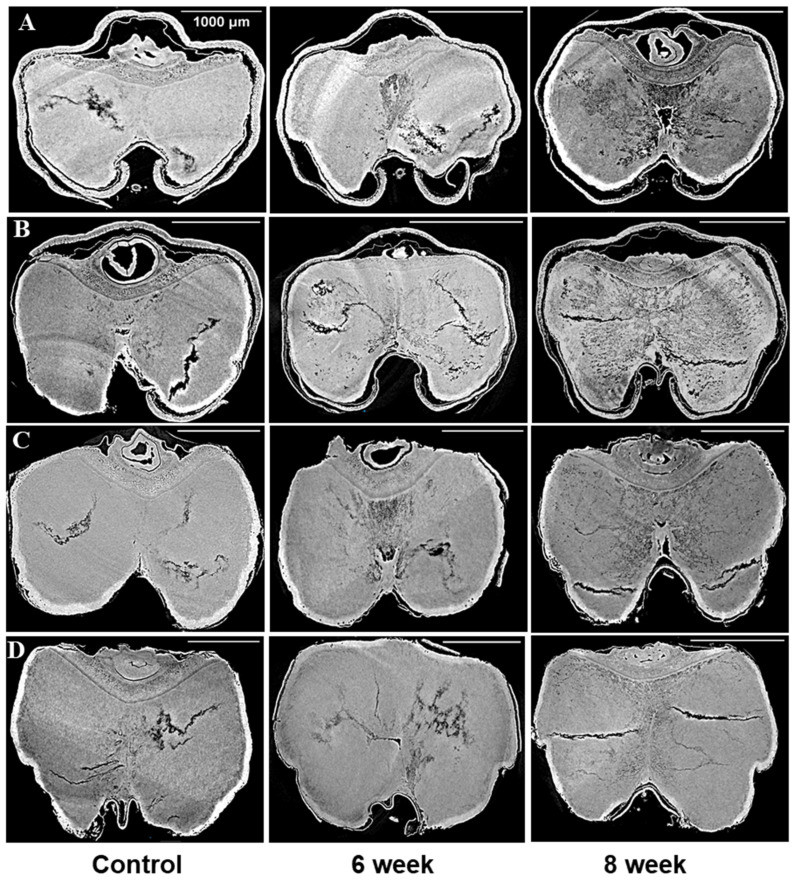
Cross sections from images of the control and 17% moisture content for four barley varieties stored for 6 and 8 weeks: (A) (6R) Tradition, (B) (2R) AC Metacalf, (C) (6Rhl) AB Cattlelac, (D) (2Rhl) Esma. The white bar in the X-ray projections represent the scalebar of 1000 µm.
